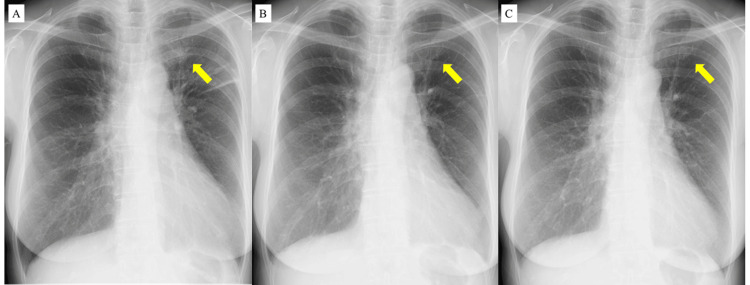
Changes of chest radiography in the clinical course TB: tuberculosis Chest radiography at the time of referral to our institution shows an infiltrative shadow in the left upper lung (yellow arrow) (A). Following the initiation of anti-TB treatment and increased dosage of oral prednisolone, the infiltrative shadow had improved. The infiltrative shadow disappeared at eight weeks after the treatment (B), and no new abnormal shadows appeared until 29 weeks (C)
